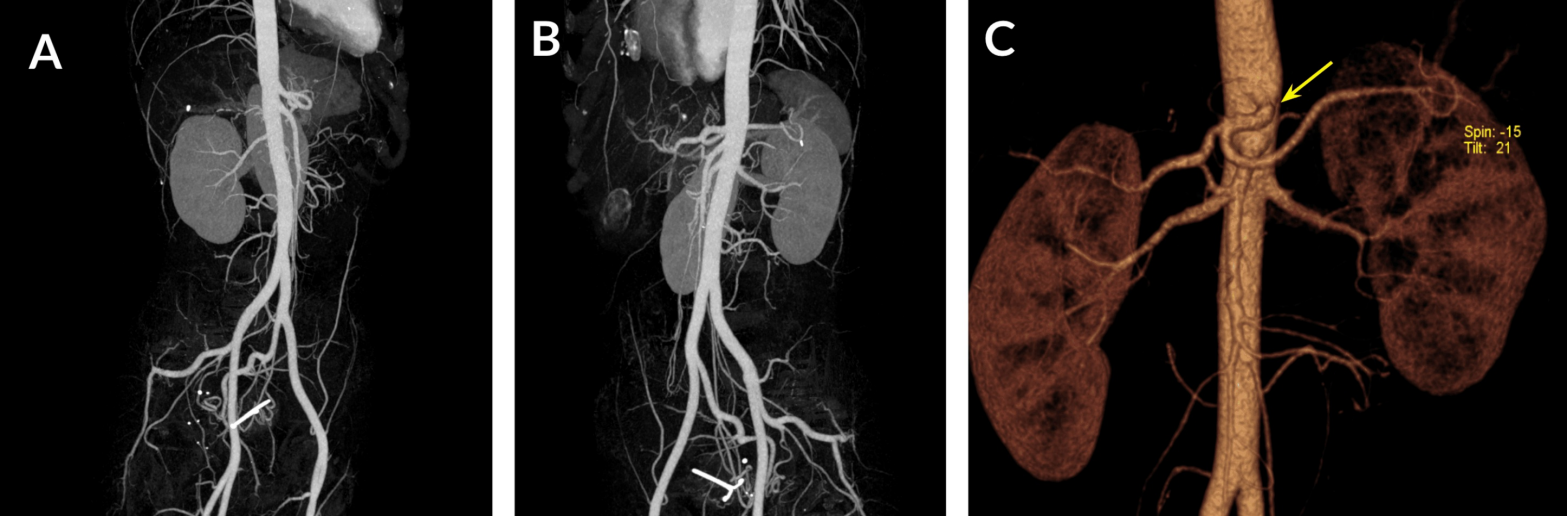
Three-dimensional reconstruction of the upper abdominal contrasted MRI. Decrease in the lumen of the caliber of the celiac artery secondary to extrinsic compression. A) Right lateral view. B) Left lateral view. C) Anterior view. Yellow arrow: filling defect in the dorsal region of the celiac trunk caused by extrinsic compression by the middle arcuate ligament. MRI, magnetic resonance imaging
